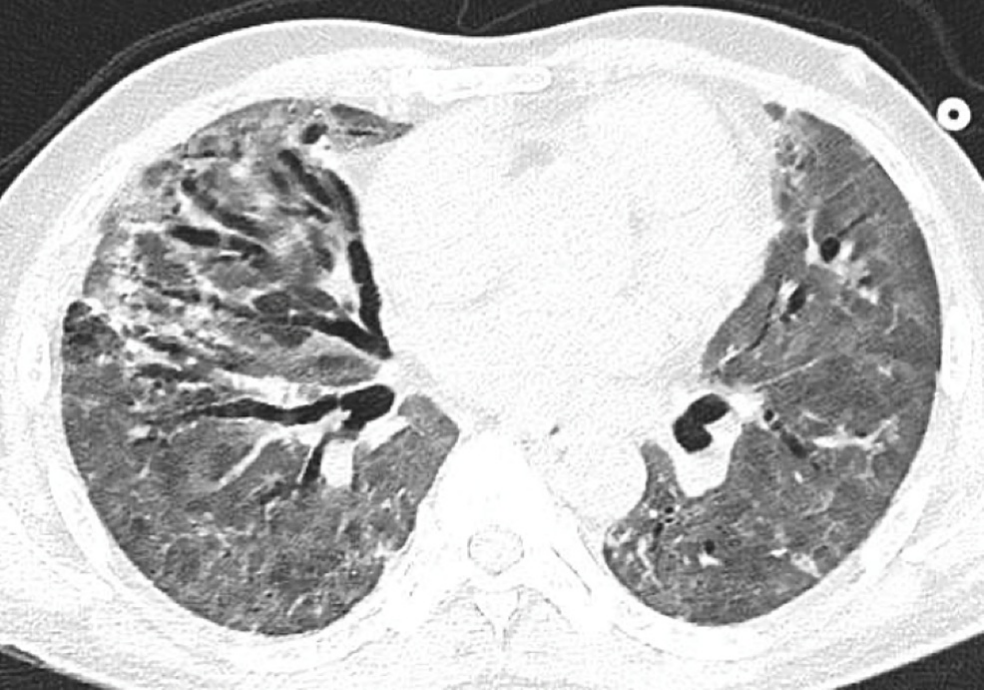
CT chest showing extensive ground-glass opacities in both lung fields with progression of the traction bronchiectasis particularly in the right lung
